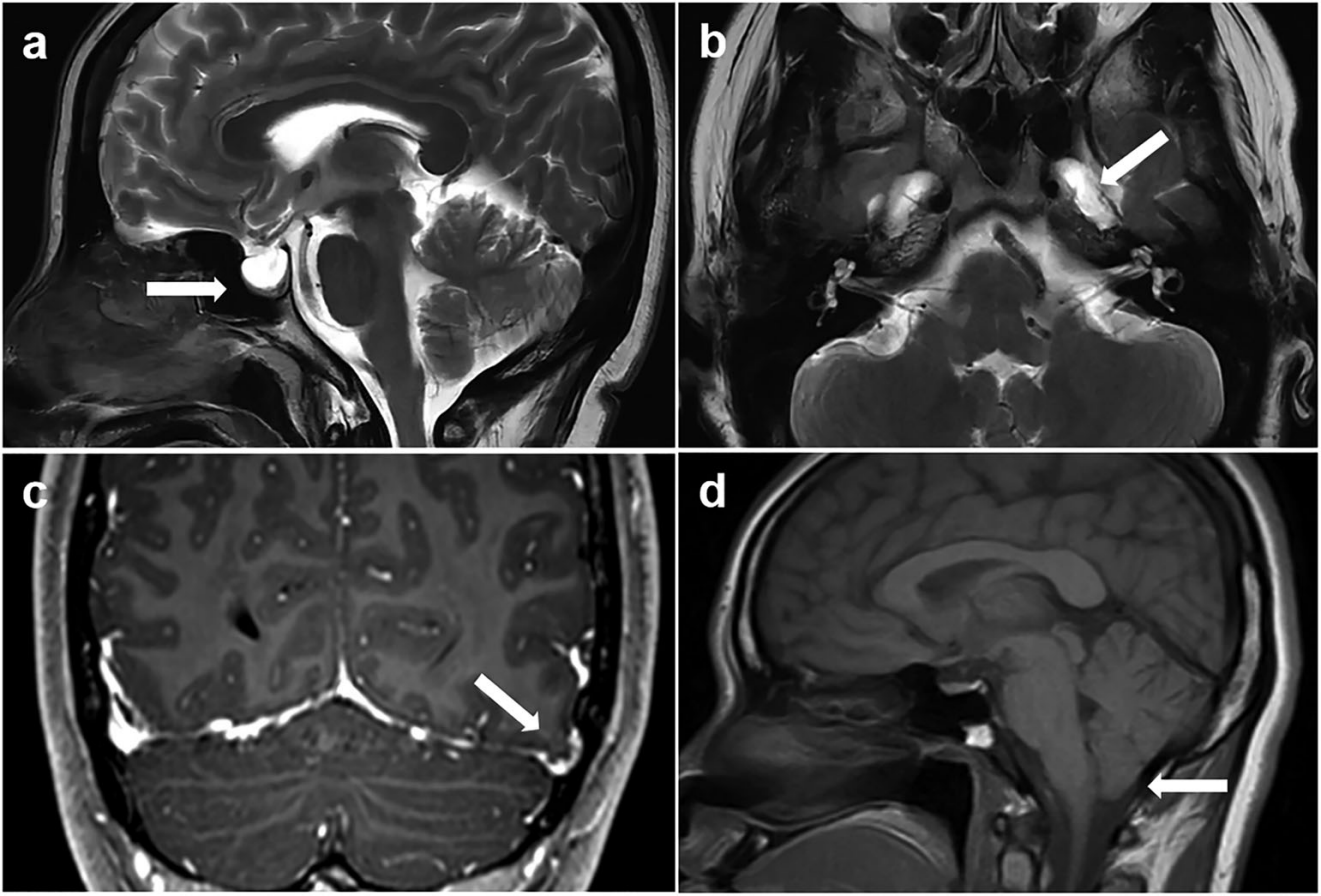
Classical magnetic resonance imaging (MRI) signs suggestive of elevated intracranial pressure. (a) Sagittal T2‐weighted MRI showing an empty sella (arrow). (b) Axial T2‐weighted MRI showing the enlarged Meckel's caves bilaterally (white arrow points to left Meckel's cave). (c) Coronal T1‐weighted postcontrast MRI showing a left temporal lobe cephalocele (arrow). (d) Sagittal T1‐weighted MRI showing mild inferior cerebellar tonsillar descent (arrow).
